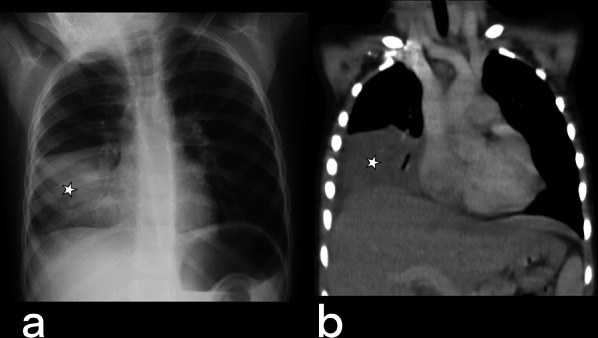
The chest X-ray (a) and coronal reformatted CT (b) image without contrast of a 4-year-old girl demonstrate unilateral pleural effusion in the right side (stars). Tuberculous pleurisy
